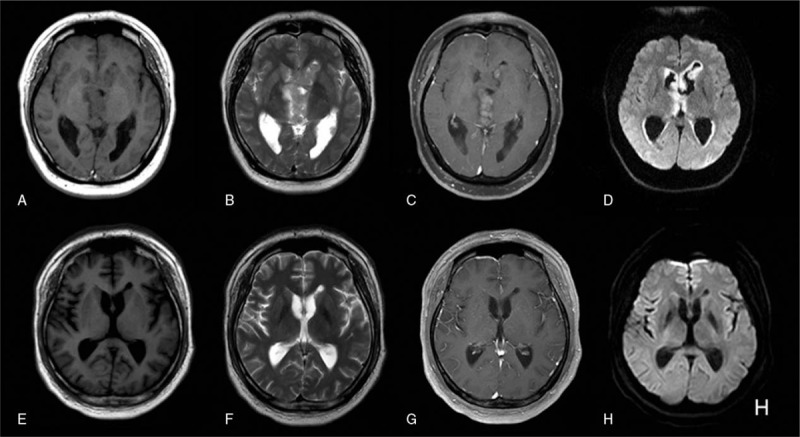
(A∼D) before radiotherapy and chemotherapy, the MRI revealed multiple tubercule on the wall of lateral and third ventricle, which were hypointense on T1-weighted images, hyperintense on T2-weighted images, and hyperintense on diffusion-weighted images (DWIs). (E∼H) After 1 month remedy, MRI showed completely disappear of the tumor.
